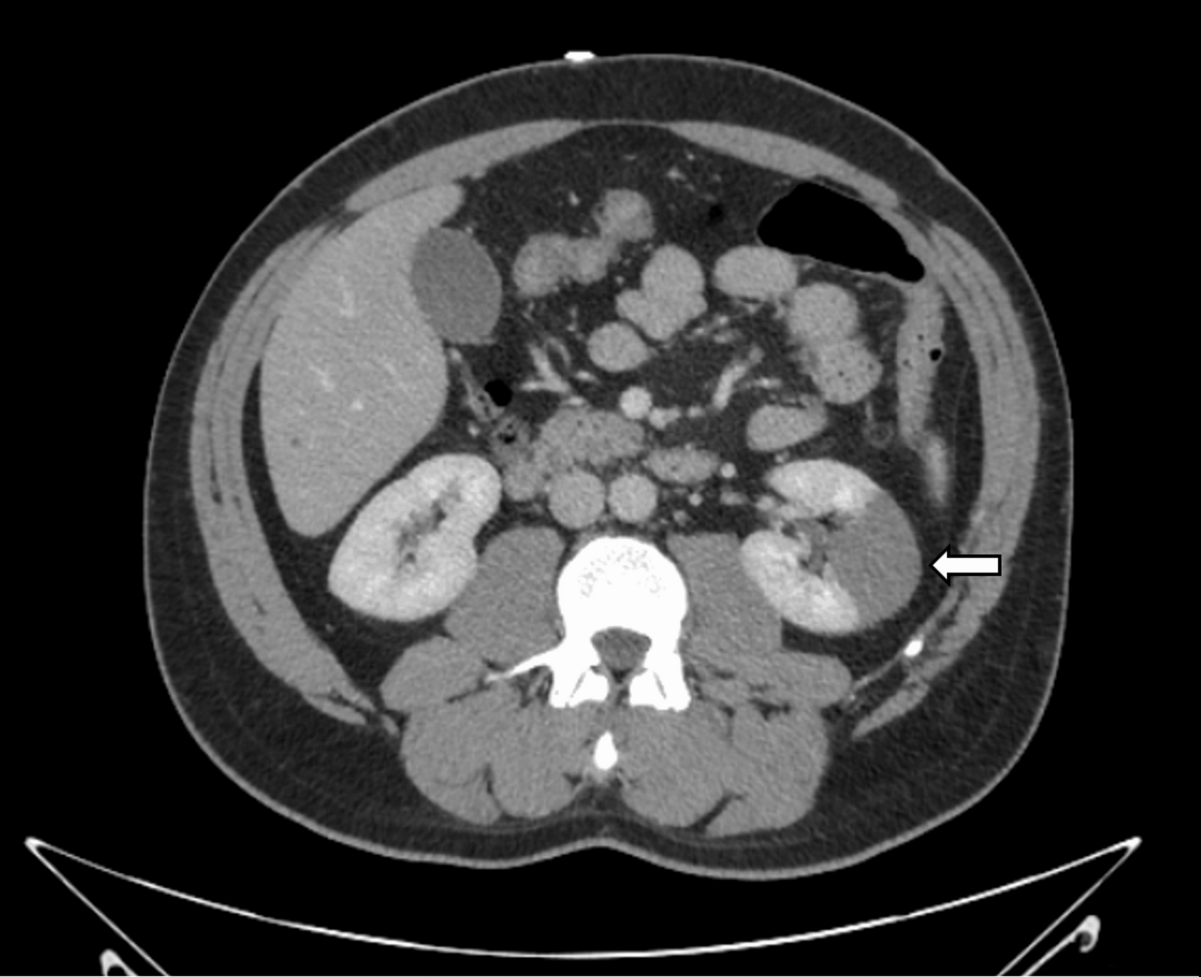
CTAP with a completely occluded segmental branch of the renal artery (white arrow) in the upper renal pole of left kidney. CTAP: computed tomography of the abdomen and pelvis
